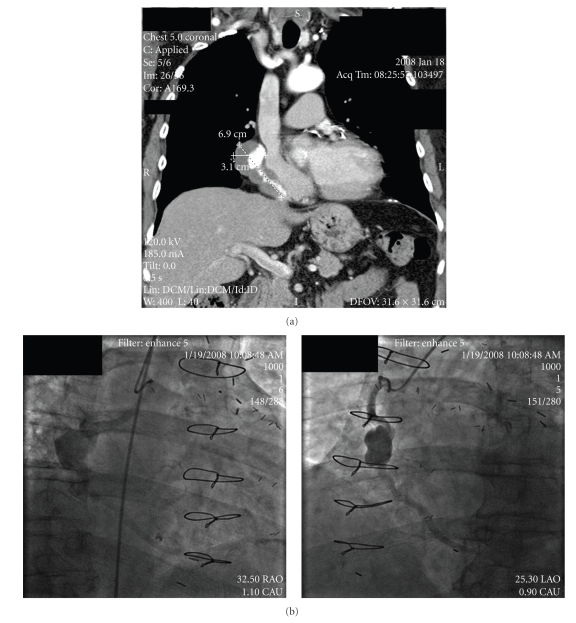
(a) Computer tomography (CT) in January 2008 of chest revealing a 3.1 cm × 6.9 cm aneurysm with contrast filling the inner lumen, and possible thrombus filling the remaining space within the aneurysm. (b) Coronary angiography depicting the aneurysm of the mid SVG, with TIMI 2 flow in right posterior descending artery (PDA) in both right anterior oblique (RAO) and left anterior oblique (LAO) views.
